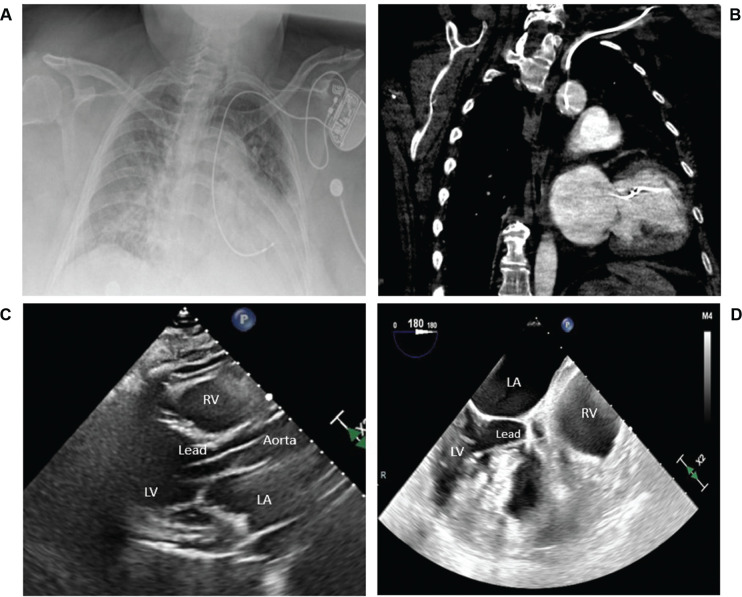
Imaging studies demonstrating a single-chamber pacemaker lead in the left ventricle, including (A) chest radiography, (B) chest computerized tomography angiography, (C) transthoracic echocardiography, and (D) transesophageal echocardiography. *Abbreviations:* LA, left atrium; LV, left ventricle; RV, right ventricle.
